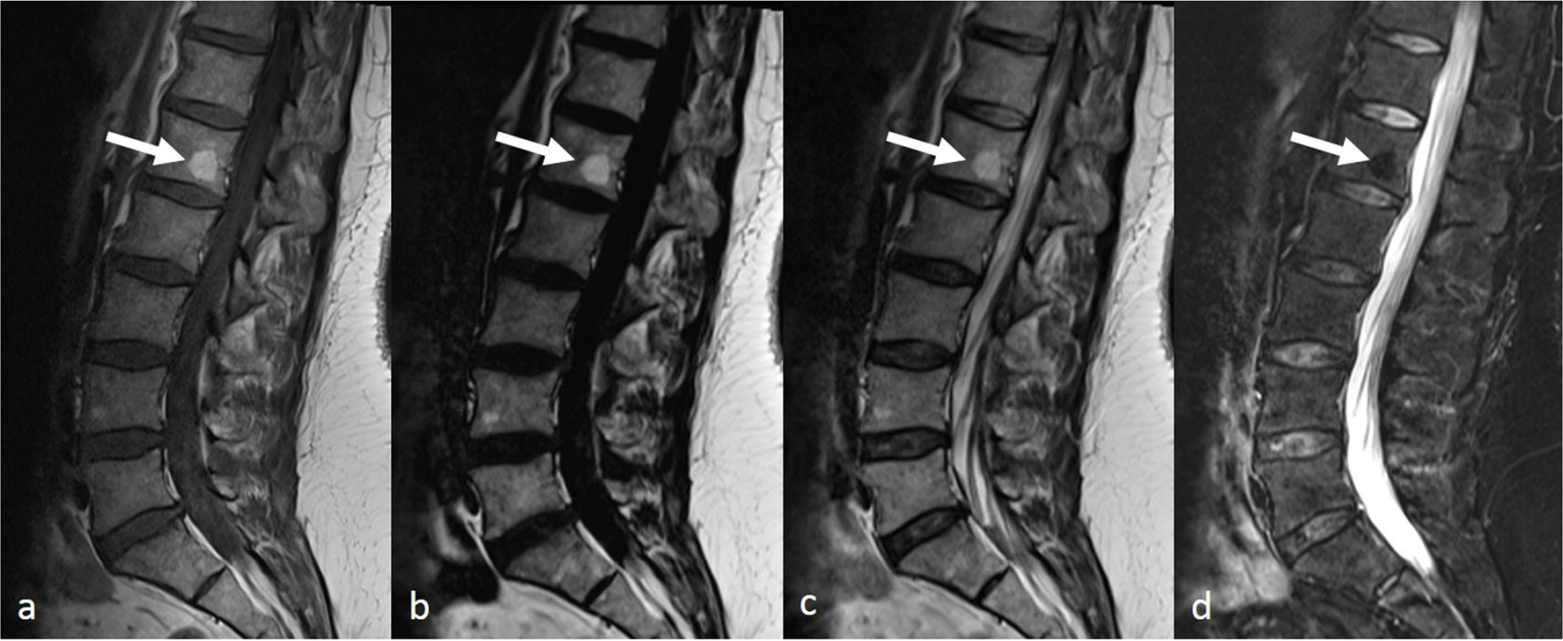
Lumbar spine MRI in a 41-year-old female including T1-weighted (a), fat-only image (b), in-phase image (c), and water-only image (d) from T2-weighted Dixon sequence. A focal hyperintense bone marrow area is visible on the L1 vertebral body on a (arrow), also clearly visible on b, with signal suppression on d, compatible with an area of focal area of red marrow depletion, due to a fatty hemangioma or a fat island
